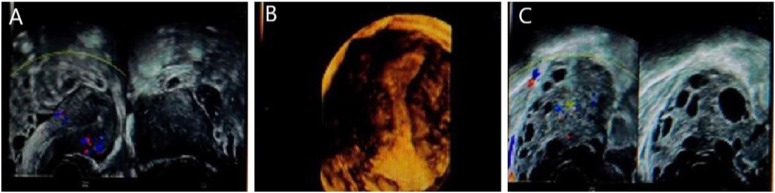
Gynecological ultrasound of patient 1. (A) represents the right ovary of the patient, with several echoless are as visible, approximately 19.5 × 19.0 mm. (B) represents uterus of the patient, and (C) represents more than 12 follicles in the left ovary, all less than 8 mm in diameter. Indicates large volume of the right ovary with multiple follicle-like echoes. More follicles in the left ovary, accompanied by pelvic effusion.
